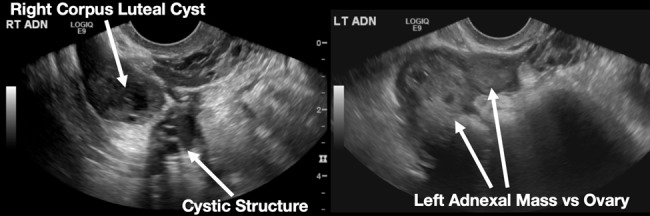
This image shows the patient’s initial ultrasound findings. The image on the right shows two arrows pointing to the left adnexa with a complex focus measuring 2.5 × 2.6 × 2.6 centimeters (cm) with a cystic focus of 0.5 cm within it and a hyperechoic thick rim. The image on the left shows the right adnexa with arrows pointing to a small, probable 0.8 cm corpus luteal cyst and an anechoic cyst measuring 1.6 cm.
